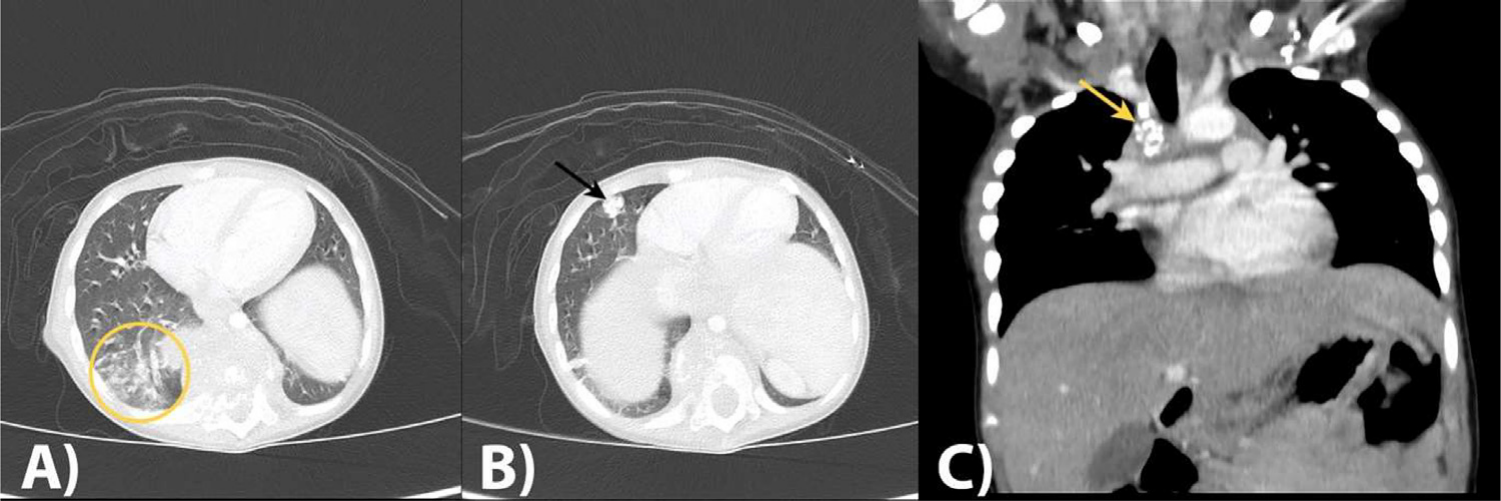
Contrast-enhanced CT scan of the thorax revealed a tree-in-bud opacity (yellow circle in A) with ground-glass opacity, along with multiple pulmonary fibrosis bilaterally in the lung window (A and B). Additionally, a calcified nodule (black arrow in B) was observed on segment 8 of the right lung, indicating the presence of tuberculoma, and mediastinal lymphadenopathy (yellow arrow in C), providing further confirmation for tuberculosis.
